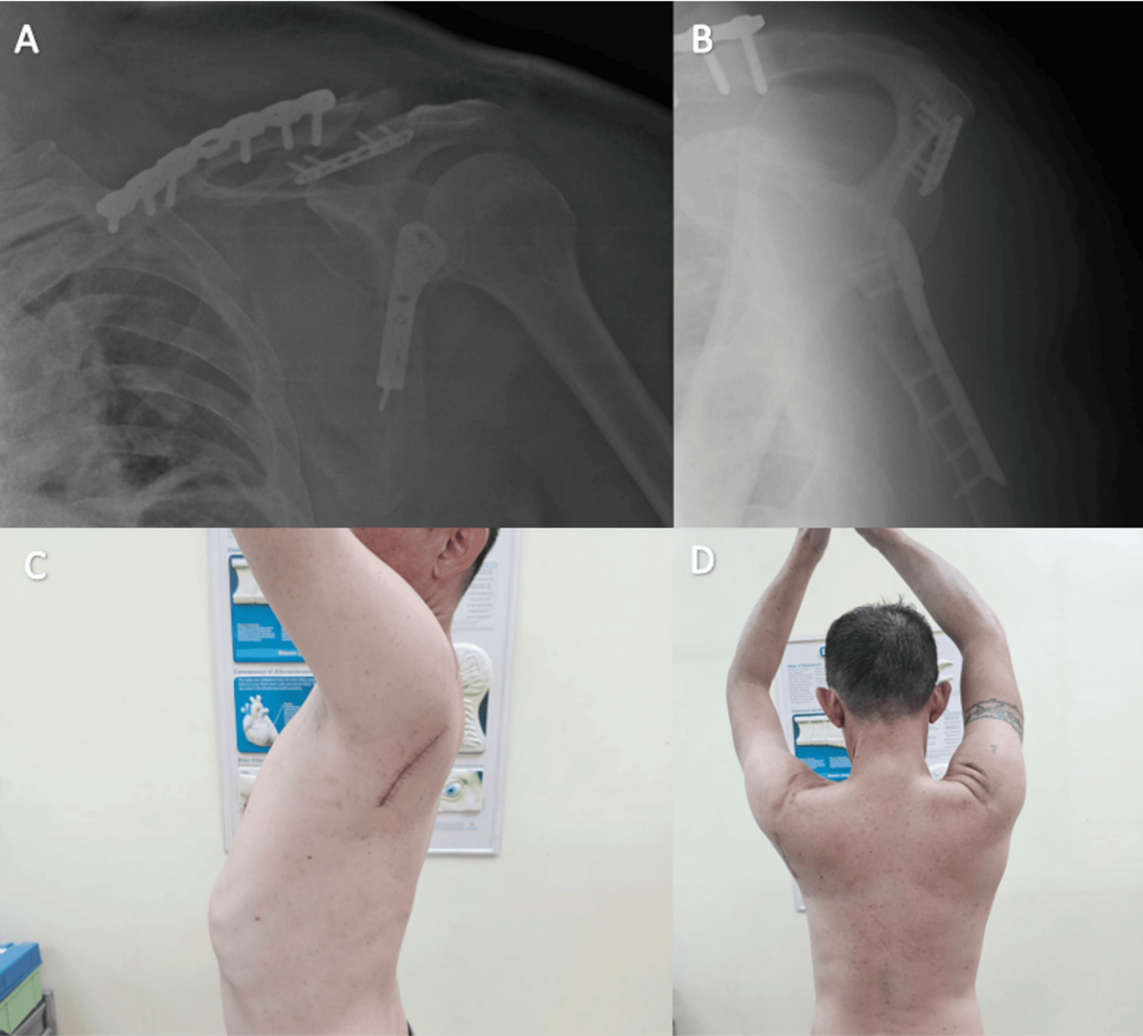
Postoperative radiograph and shoulder range of motion A: Radiograph (anterior-posterior view)
